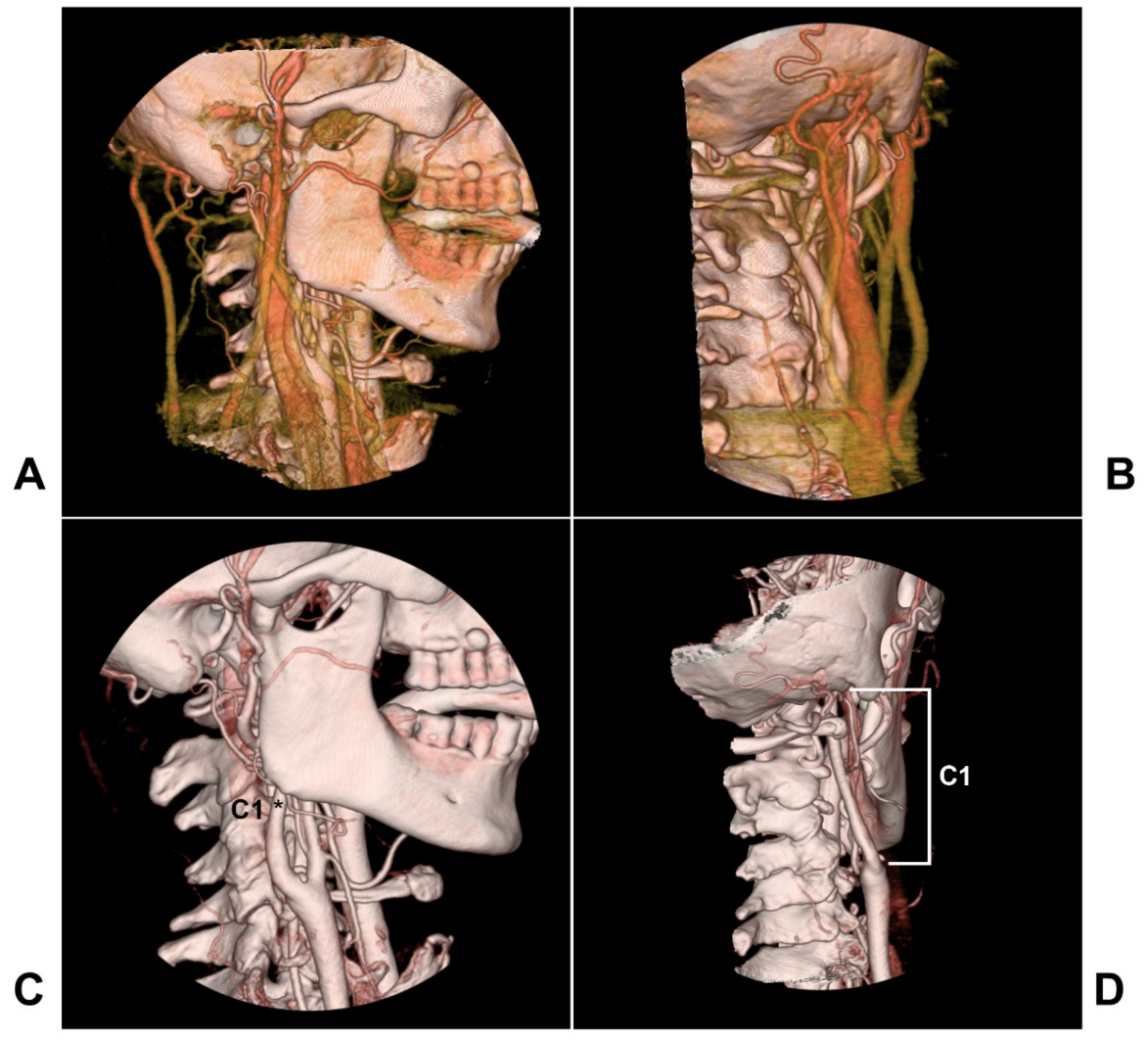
The anatomy of the extracranial ICA region. A-B: Lateral view (A) and posterior anterior view (B) of CTA showing carotid veins around the extracranial ICA. C-D: Lateral view (C) and posterior anterior view (D) of CTA showing that the extracranial ICA extends from the bifurcation to the skull base, and the extracranial ICA belongs to the C1 segment of the Bouthiller classification (asterisk). Abbreviations: CTA: computed tomography angiography; ICA: internal carotid artery
